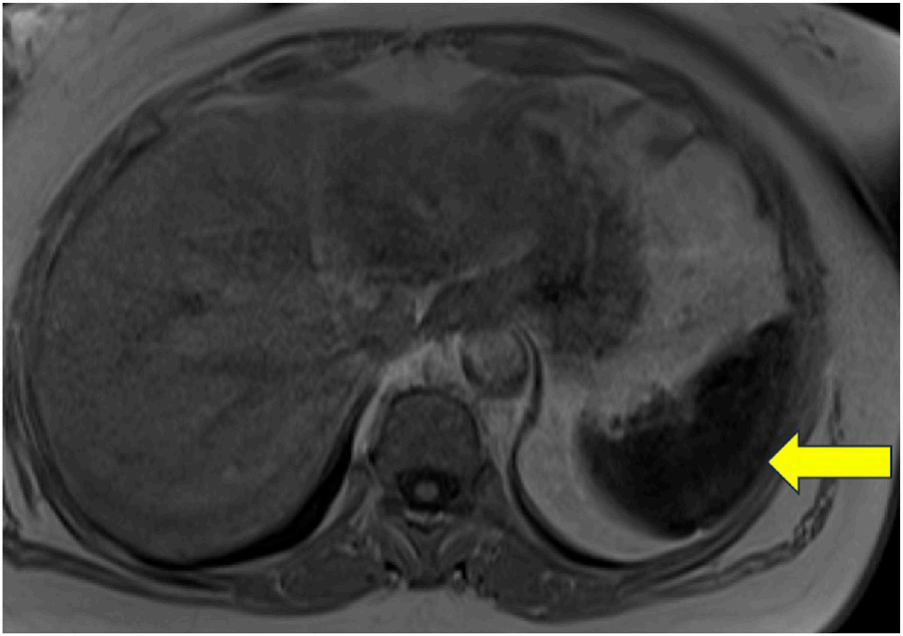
Non-contrast MRI of the abdomen of a patient with Gaucher disease and iron overload. This image represents a T1-weighted image with a uniformly hypointense spleen, which was associated with a signal dropout on the in-phase gradient echo sequence with a longer time to echo (TE), suggestive of diffuse iron deposition.
